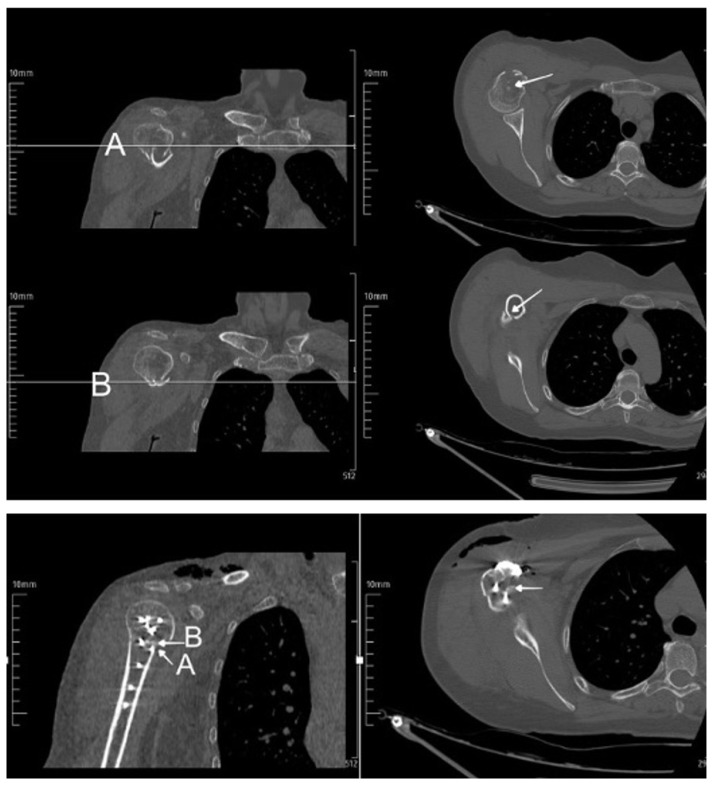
Pre- and postoperative measurement of medial hinge impaction on computed tomography (measured as the distance between the projections of the topmost slice, where the distal part (diaphysis) of the fracture is seen in the axial plane, and the lowest slice, where the proximal part (humeral head) of the fracture is seen in the axial plane, on the mapped coronal slice).
